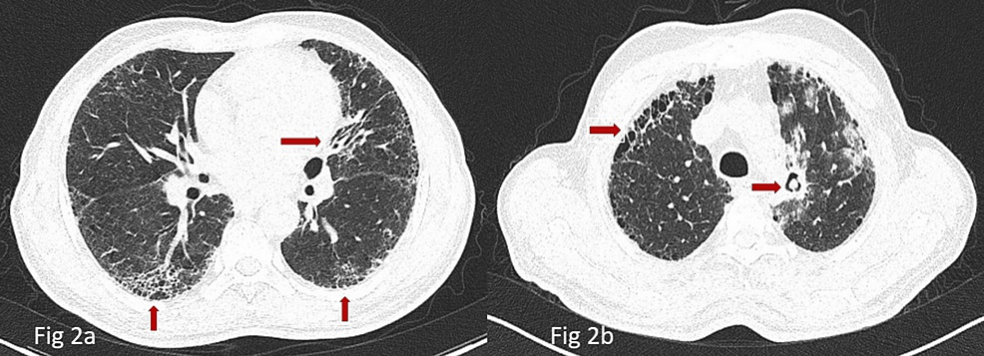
Aspergilloma in a patient of idiopathic pulmonary fibrosis (a) Computed tomography of the chest (parenchymal window) shows bilateral basal and subpleural honeycombing (Upward arrows) consistent with idiopathic pulmonary fibrosis. Also appreciable is tractional bronchiectasis in the left hilar region (horizontal arrow). (b) Computed tomography of the chest (parenchymal window) at a section above the carina shows a cavity in the left hilar region, apparently filled with a fungal ball highly suggestive of aspergilloma (right horizontal arrow). Also noticeable is subpleural honeycombing mixed with cystic air spaces in the periphery (left horizontal arrow).
